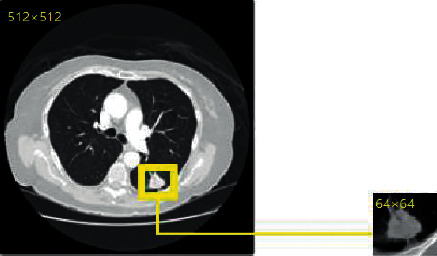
Example of original CT image reduction.
